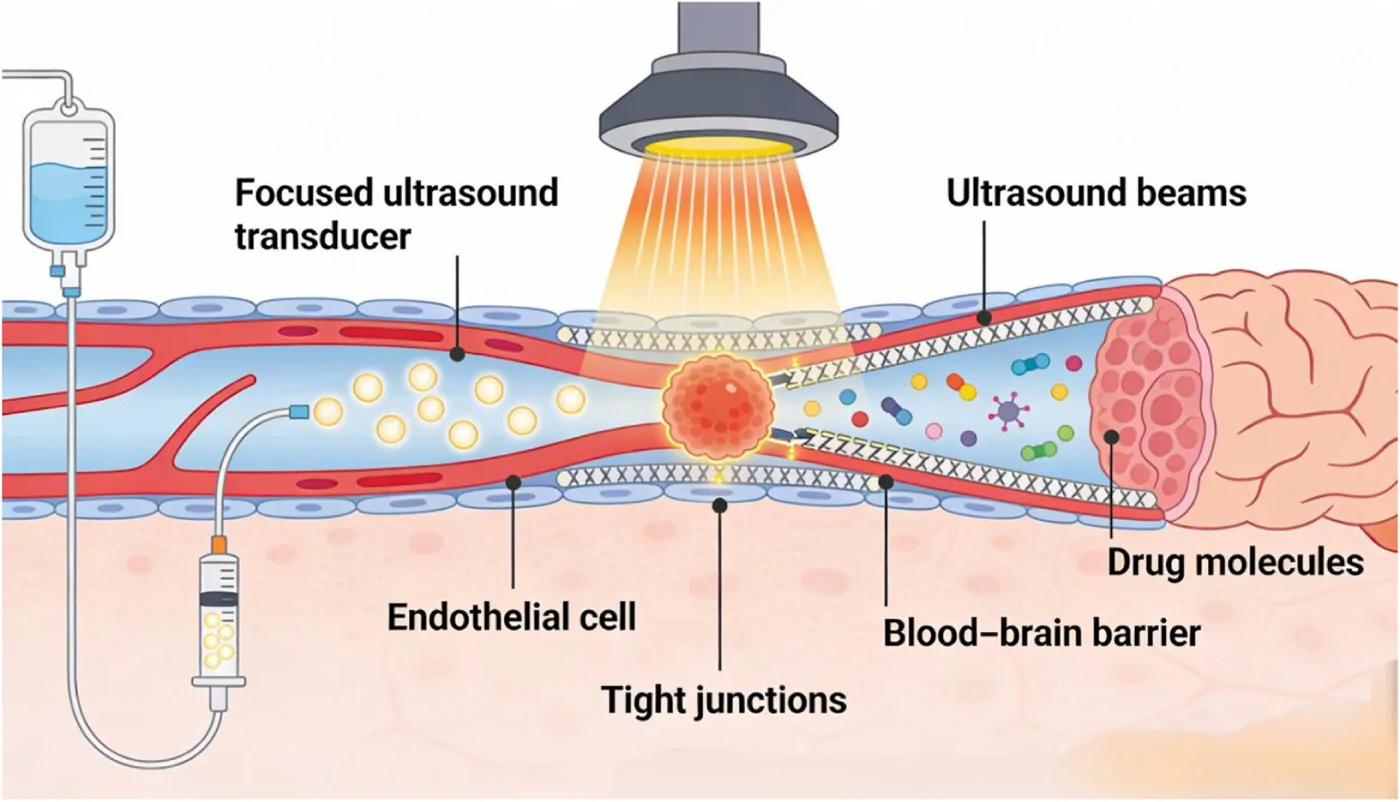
Focused ultrasound–mediated modulation of intracranial lesions.
